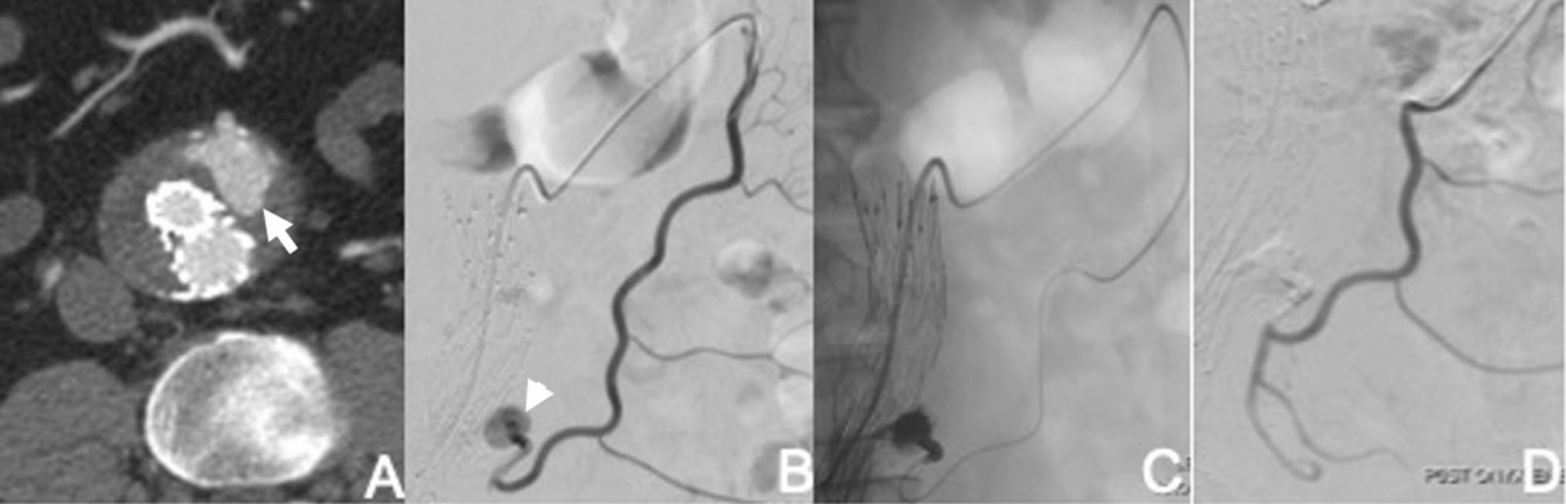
Transarterial embolisation of type 2 endoleak. A Axial CT image shows endoleak arising close to origin of IMA (arrow). B Angiogram via microcatheter placed into middle colic branch of SMA confirms endoleak (arrow head). C Microcatheter passed into endoleak cavity via the left colic branch of the IMA, and embolisation performed with Onyx. D Completion angiogram shows no further filling of endoleak
